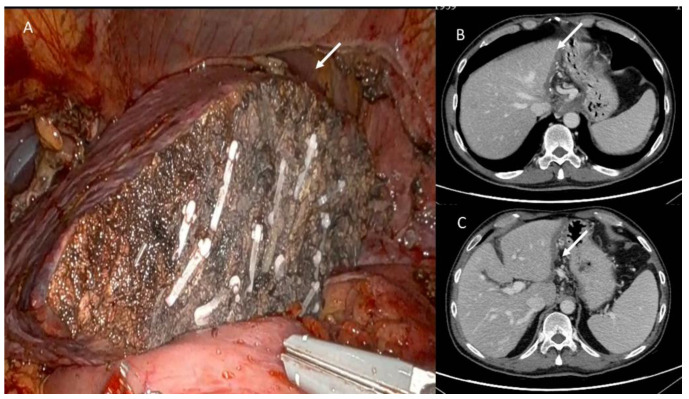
Robotic Anatomycal S2 segmentectomy in patient with HCC (A). In (B,C), CT assessment at 3 months follow-up: arrows show scar tissue.
